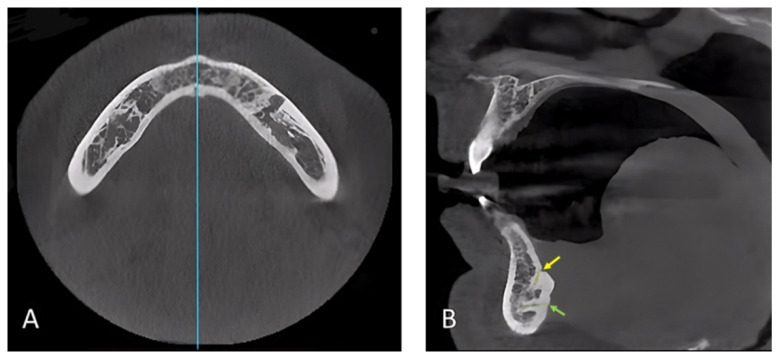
CBCT scan orientation. (A) Axial cut at the level of the genial tubercle to orient the midline. (B) Sagittal cut at the midline showing the mandibular lingual canals. The yellow arrow points to the supra-spinosum foramen, which is the opening of its corresponding canal (i.e., yellow line). The green arrow points to the infra-spinosum foramen, which is the opening of its corresponding canal (i.e., green line).
